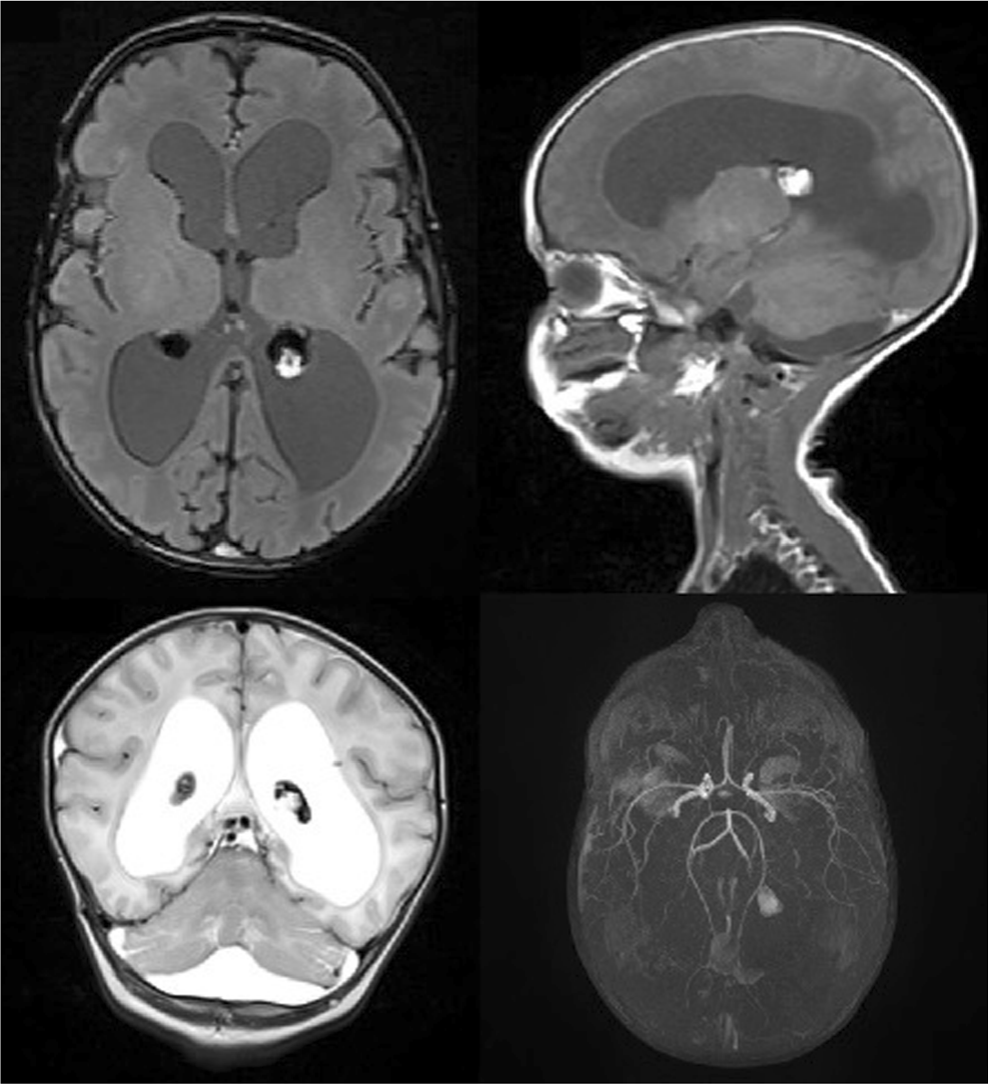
Brain magnetic resonance imaging of the neonate reported as case 2. Hydrocephalus and posthemorrhagic changes in both choroid plexuses, in the left choroid plexus fresh bleeding focus
